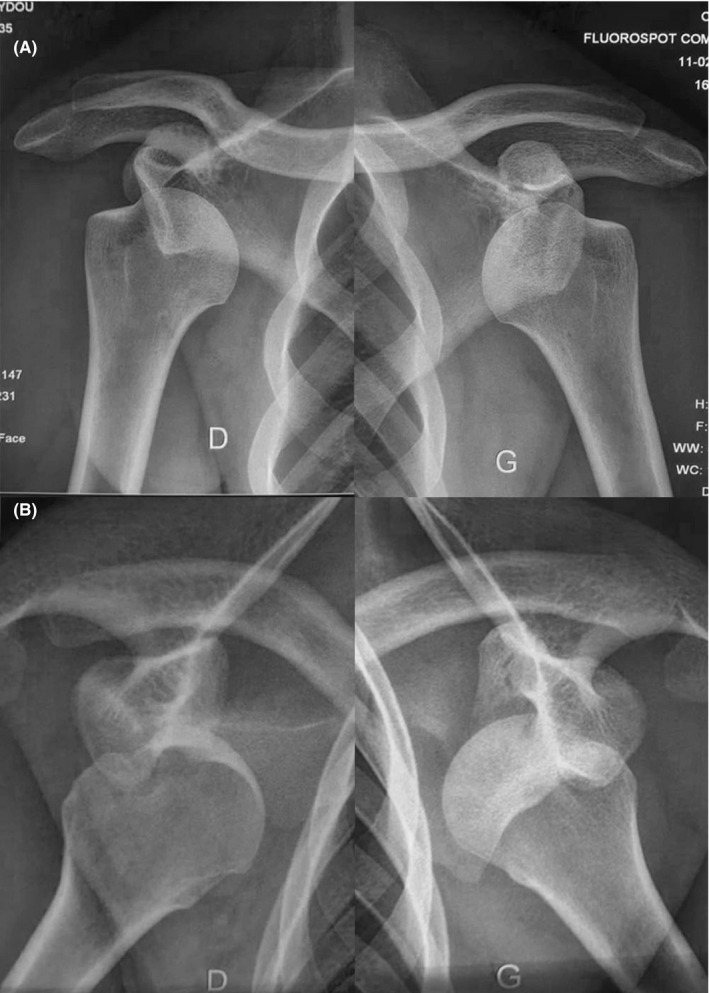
Shoulder radiographs (Case 1). AP (A) and Bernageau (B) views showed anteromedial subcoracoid displacement of both humeral heads
